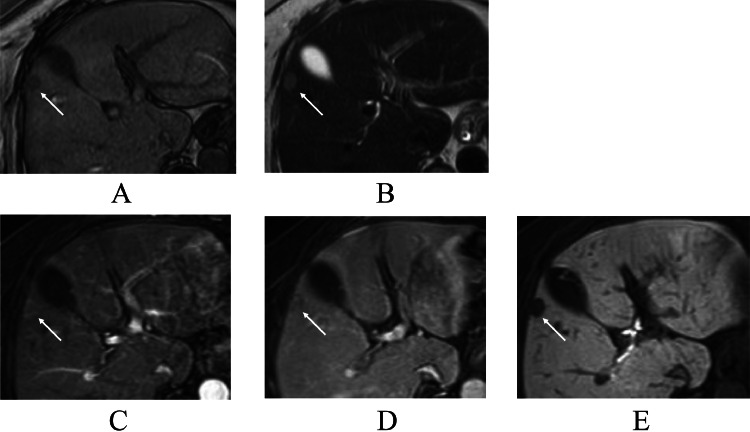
Magnetic resonance imaging (MRI) findings. MRI of the tumor (arrows) showed low signals on T1-weighted images (A), faint high signals on T2-weighted images (B), nominal enhancement both on early and late phase images (C and D), and very low signals on hepatobiliary phase images (E).
